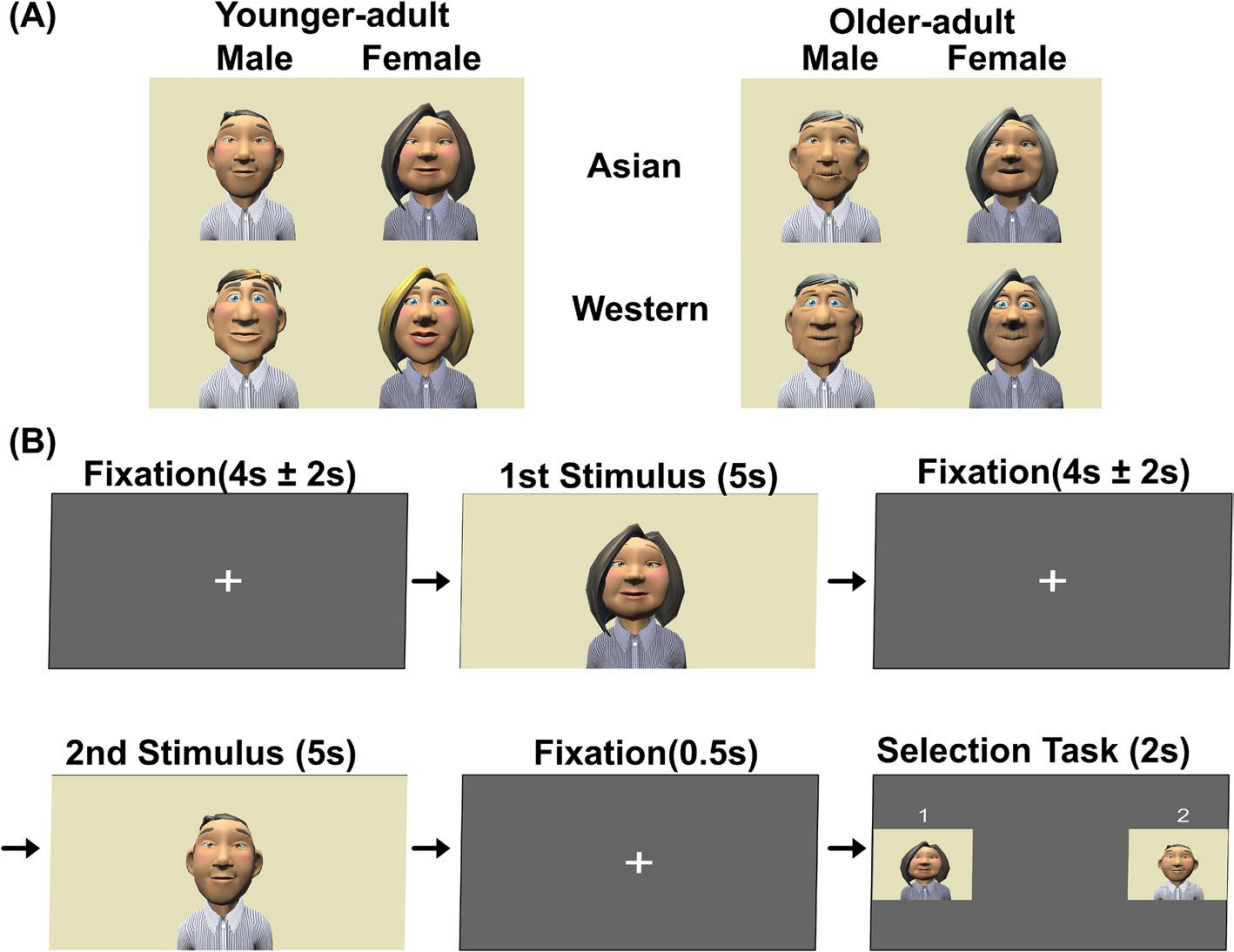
Experimental procedure in MRI scanner. Virtual avatars were created by Toon people ver 4.0 which is a Unity asset produced by JBGarraza CC BY 4.0.
